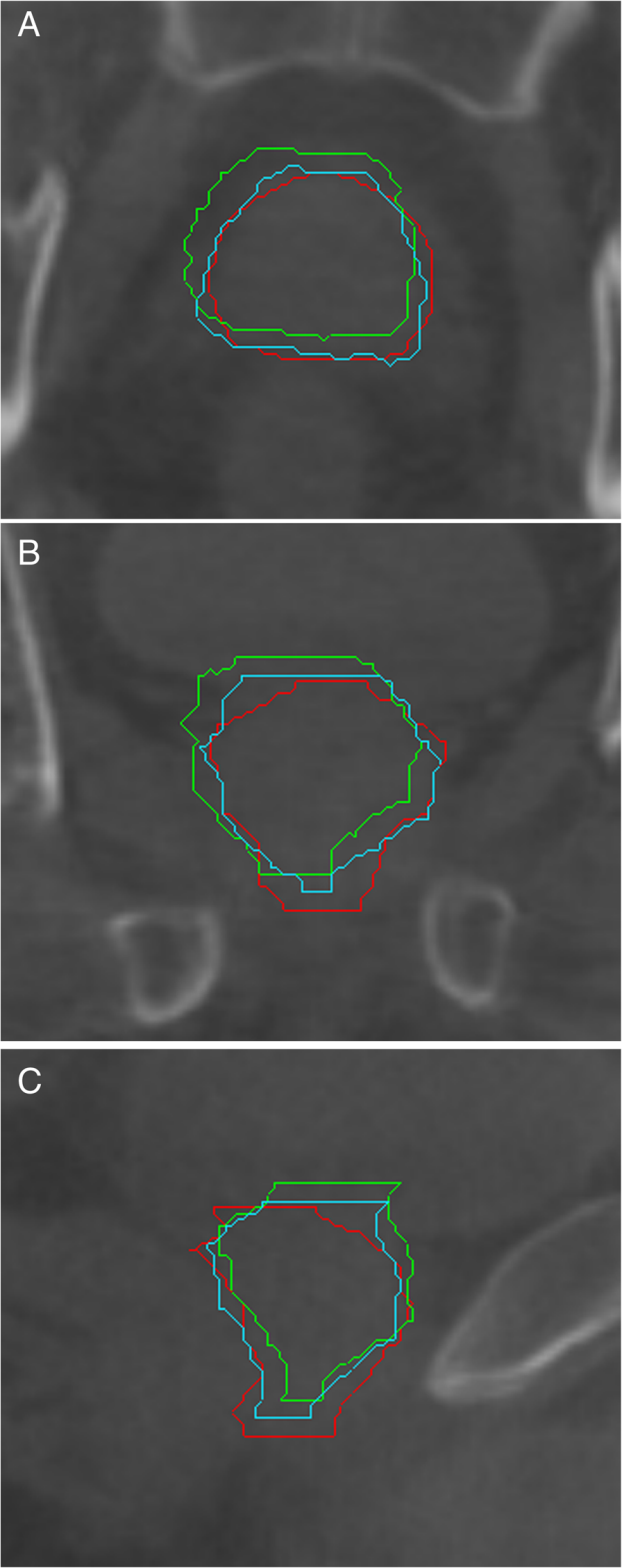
Example of contours. Example of (red) manual and automatic prostate contours produced by (green) bony and (blue) 8-mm local RRs displayed on top of the corresponding CBCT image in the (A) axial, (B) coronal and (C) sagittal planes.
